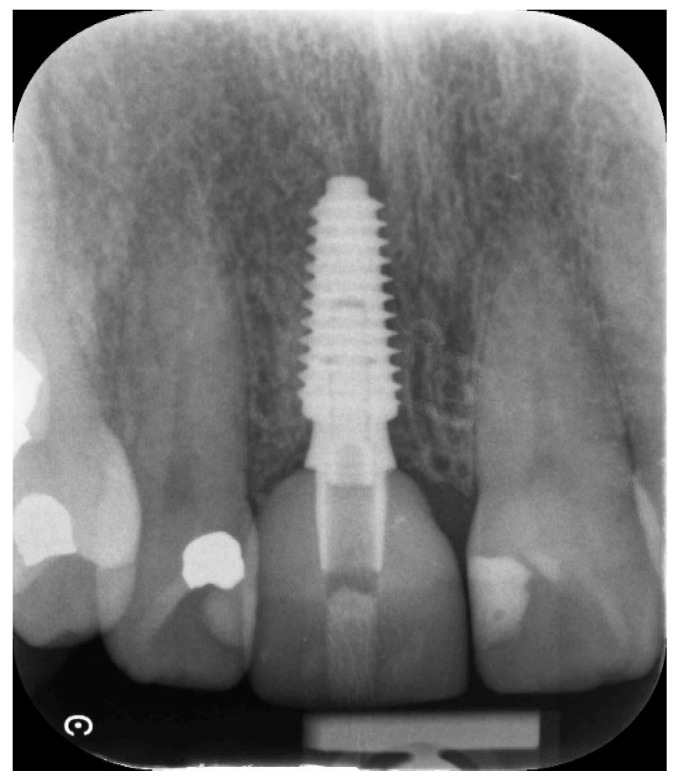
A Megagen Anyridge^®^ implant was placed at 8 weeks after ARP. The implant bed was soaked with an i-PRF solution using a disposable syringe just prior to the insertion of the implants. The periapical radiograph shows good implant integration and maintenance of the newly generated bone within the grafted extraction site at UR1 at a follow-up appointment.
